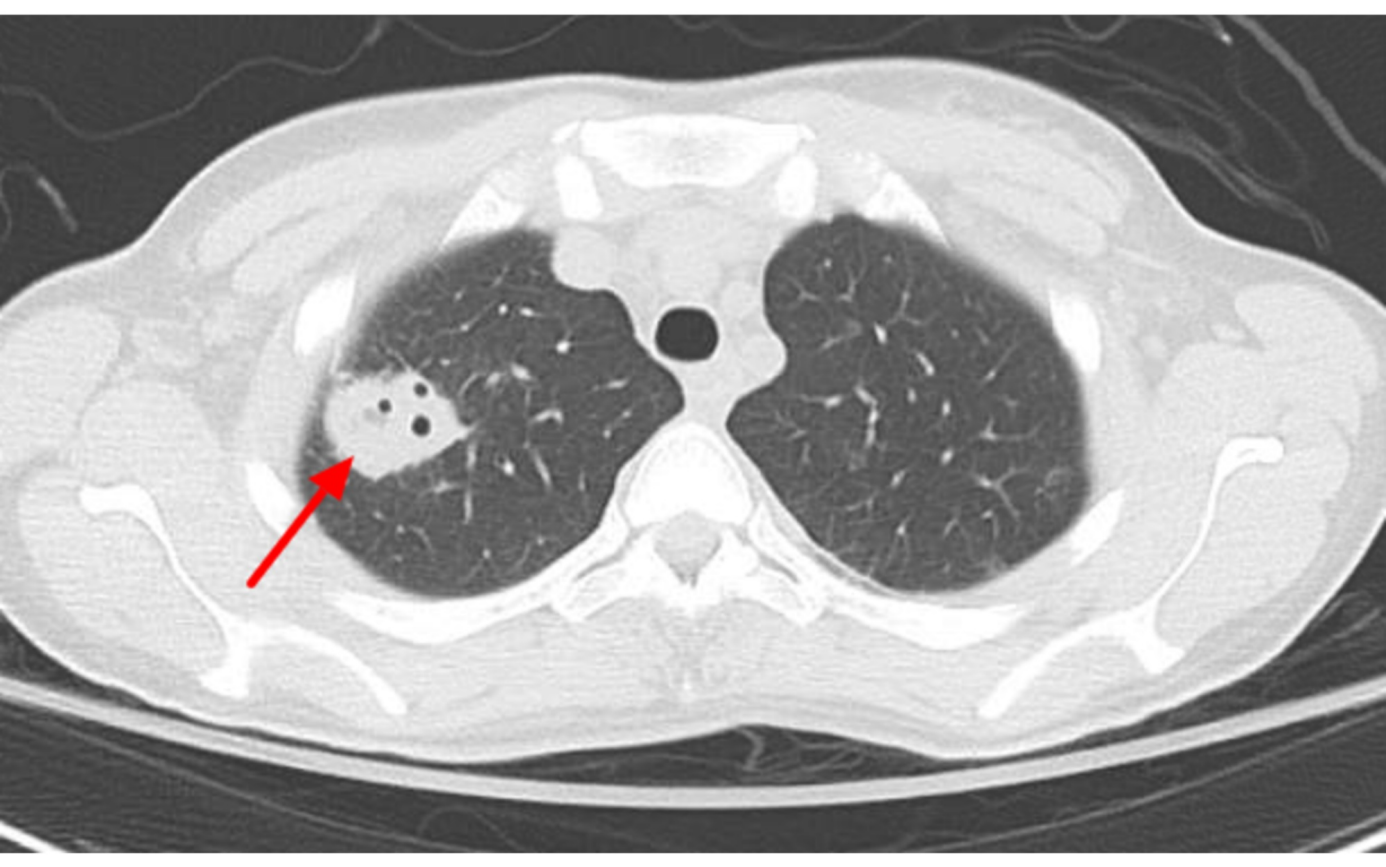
Non-contrast chest CT showed cavitary lesions (red arrow) on the right lung (largest ~3 cm) suggestive of septic emboli.
